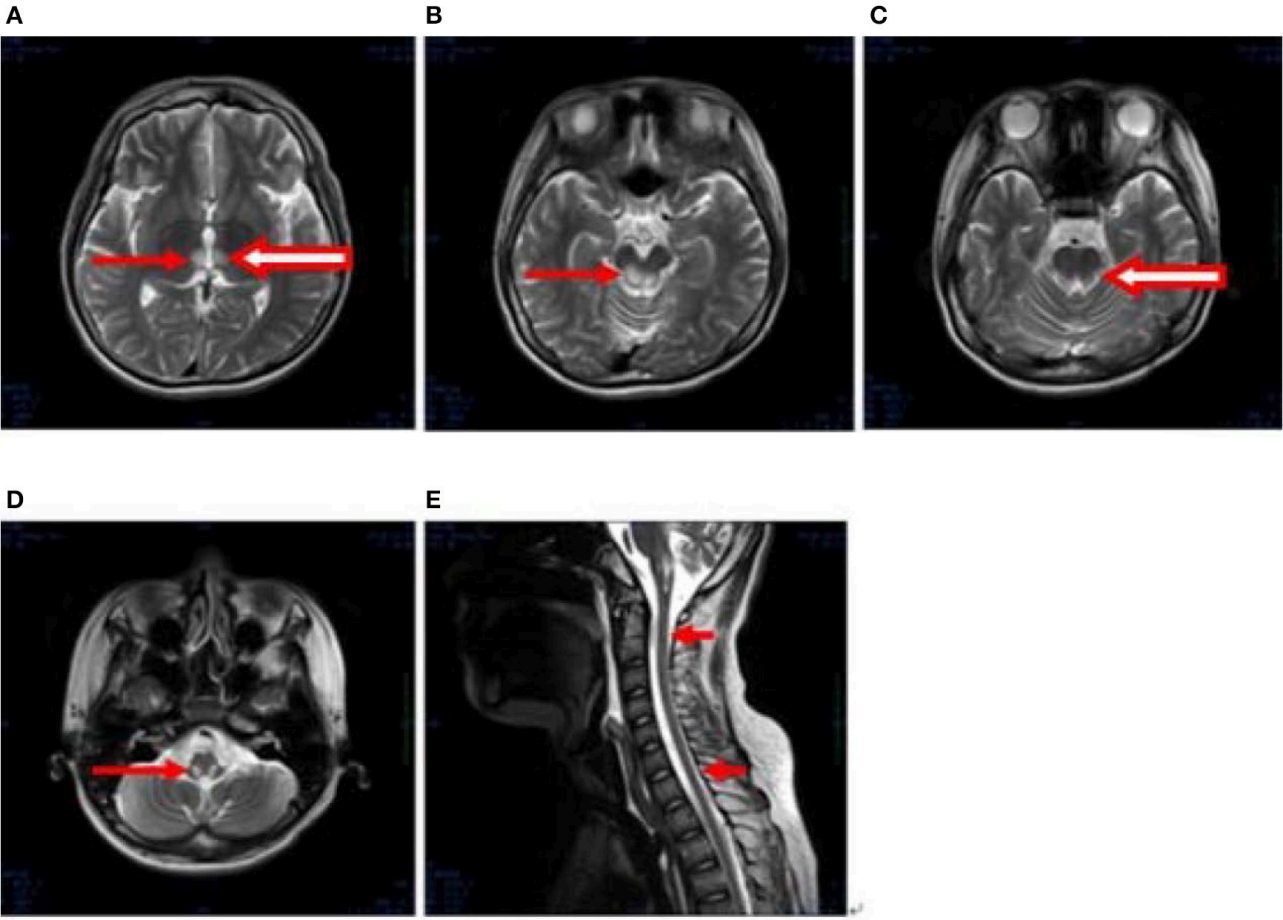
(A–E) Axial, T2-weighted MRI at 2 months following hospitalization revealed hyperintensities in the bilateral thalamus (arrow and hollow arrow in A), tegmental area of the midbrain (arrow in B), pons (hollow arrow in C), and medulla (arrow in D). The thalamic lesions had increased in size, and new lesions had emerged (hollow arrow in A,C). There were no evident lesions in the cervical and thoracic spinal cord at this stage (small arrows in E).
